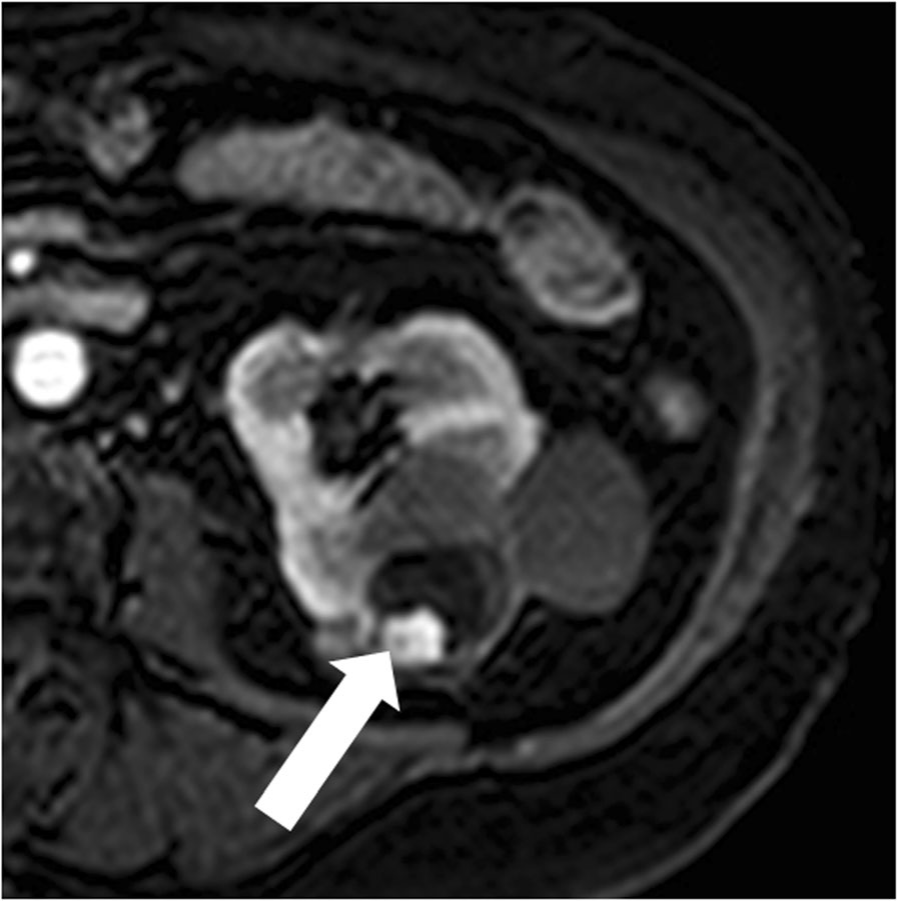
Bosniak category IV renal cyst. Axial contrast-enhanced T1-weighted MR image shows a lesion with a peripheral, enhancing, nodule (arrow)
